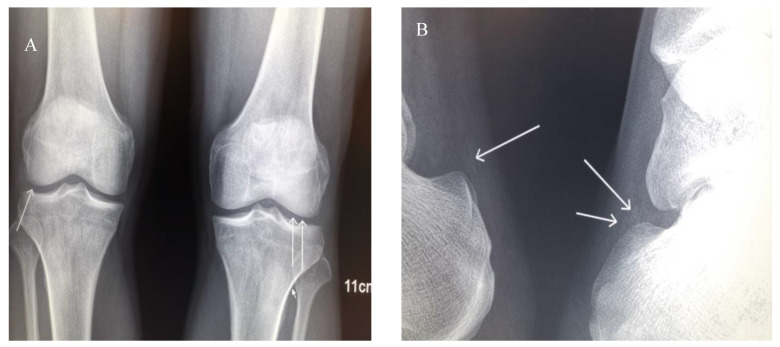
X-ray of joints. (A) Anteroposterior projections of the patient’s knees. Extensive chondrocalcinosis of the fibrocartilage of the medial and lateral menisci (arrows) is present. (B) Lateral projections of the ankles. Calcification of the Achilles tendons is present.
